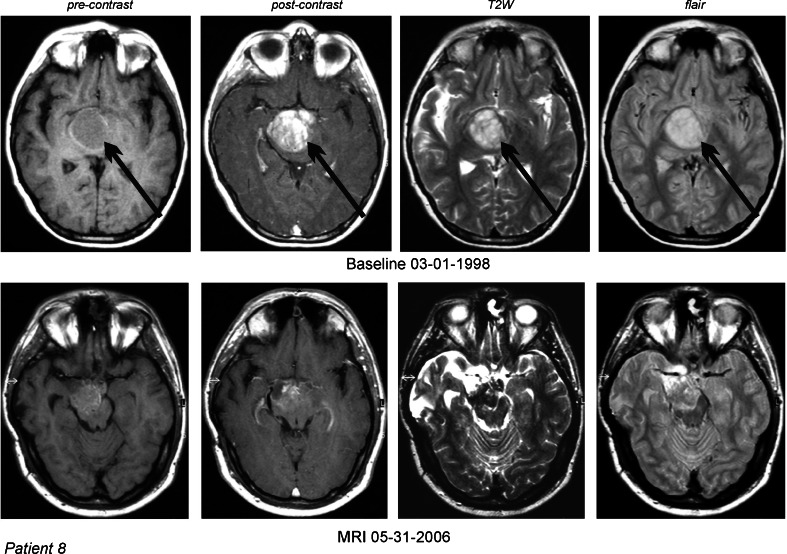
DIPG in a 10-year-old male (case 8) which recurred two times after partial surgical resection. MRI of the head: 1—T1 nonenhanced, 2—contrast-enhanced, 3—T2W, and 4—FLAIR images. PR was documented by the MRI and CR was established by the normalization of the follow-up PET scans. *Arrows* indicate tumors
